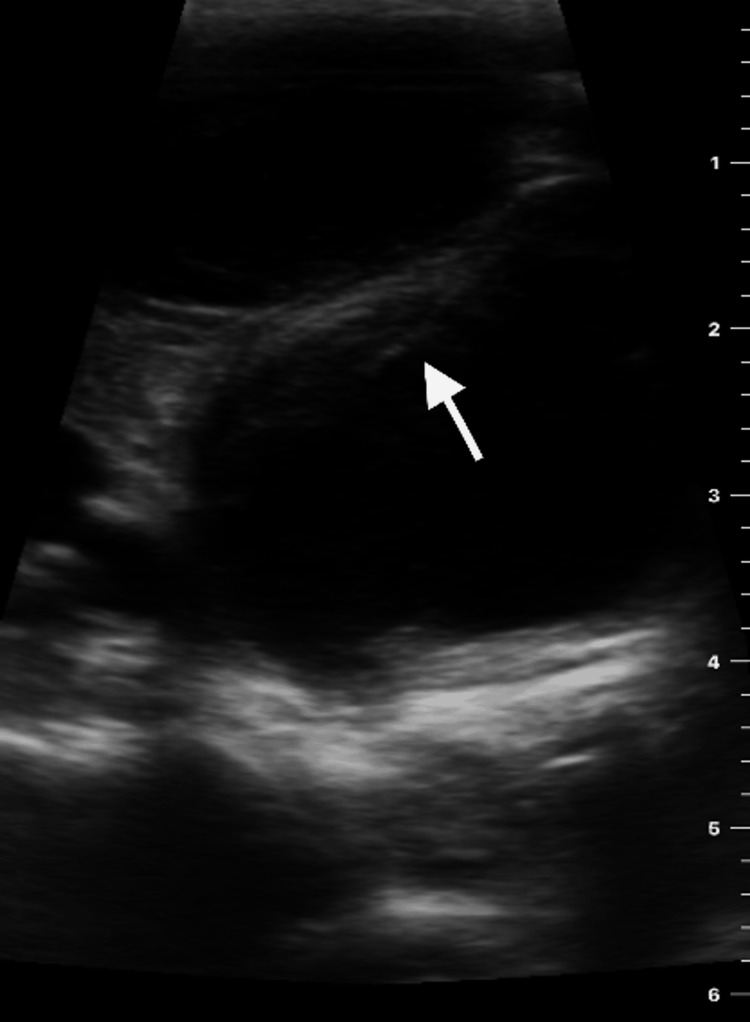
Bedside ultrasonography of the right-sided neck mass demonstrating a superficial, multicystic lesion with internal septations. The arrow indicates a septation between adjacent hypoechoic cystic components, consistent with a complex cystic mass in the right lateral neck
